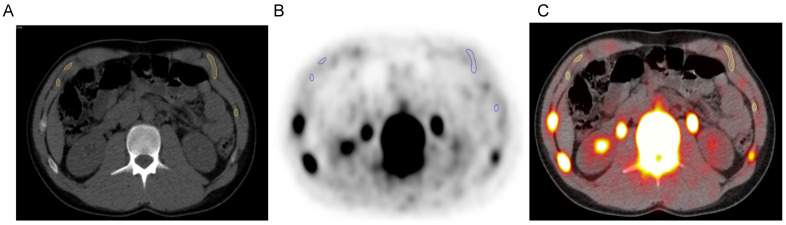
Regions of interest (ROIs) in the costal cartilage. Representative image of the ROI delineated on the axial (A) CT, (B) PET, and (C) fused PET/CT images. The outline of the cartilage is highlighted by the yellow or blue contours, drawn using manually generated CT-based segmentations.
